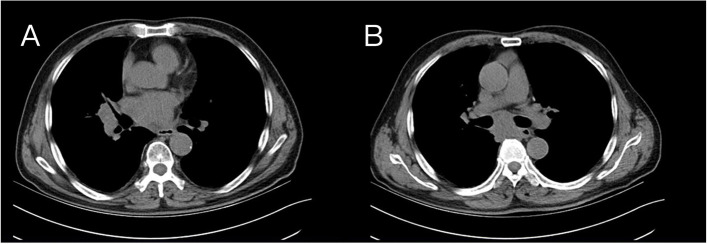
The chest CT imaging of lung metastasis in April 2021 before tislelizumab administration. A The enlargement of the right hilar lymph nodes. B The swollen subcarinal lymph nodes in the left upper lobe
